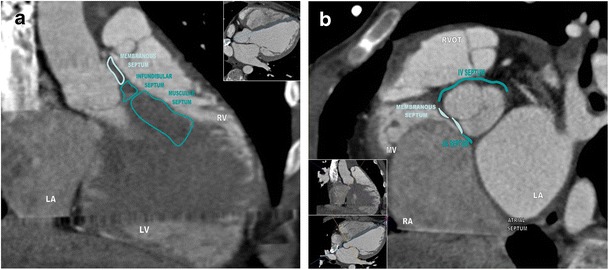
a and b: Anatomy of the interventricular septum. a Parasternal long-axis view of three portions of the interventricular septum: interventricular membranous septum, infundibular septum, and trabecular or muscular septum. b Parasternal short-axis view of the cardiac septum: atrialseptum, atrioventricular septum, and the membranous portion of the interventricular septum are shown
